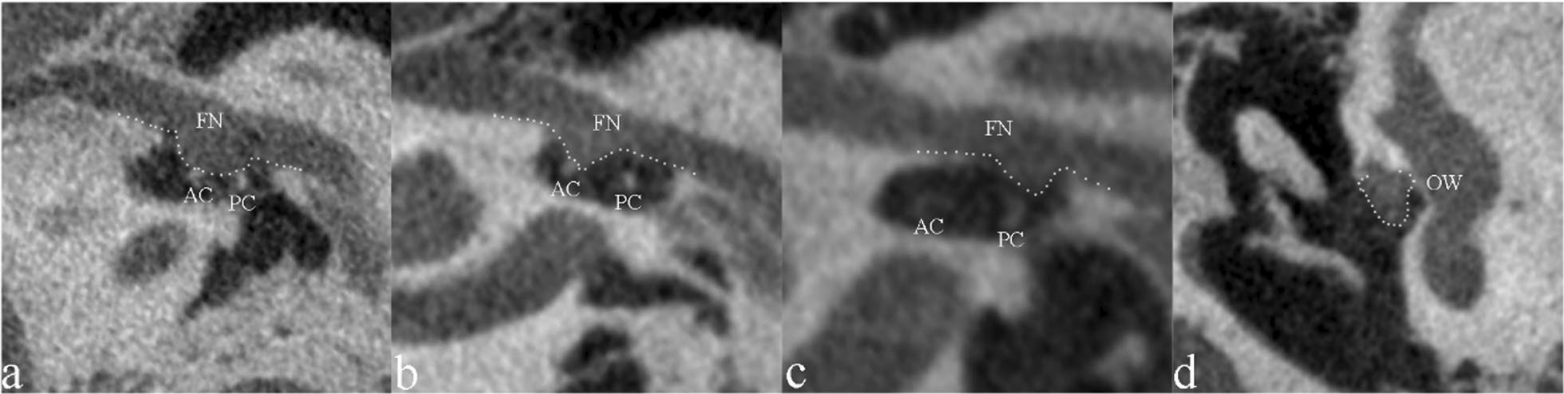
Imaging morphology of overhanging facial nerve (FN) on U-HRCT images. a Oblique sagittal image of a 20-year-old woman showing the entire course of the right FN near the oval window protruding downwards (dotted line), touching the anterior crus (AC) and posterior crus (PC) of stapes. b Oblique sagittal image of a 35-year-old woman showing the anterior portion of the right FN near the oval window protruding downwards (dotted line), touching the AC of stapes. c Oblique sagittal image of a 40-year-old man showing the posterior portion of FN near the oval window protruding downwards (dotted line), close to the PC of stapes. d Coronal image of the case shown in image B showing irregular morphology and downward protrusion of FN, blocking the oval window (OW)
